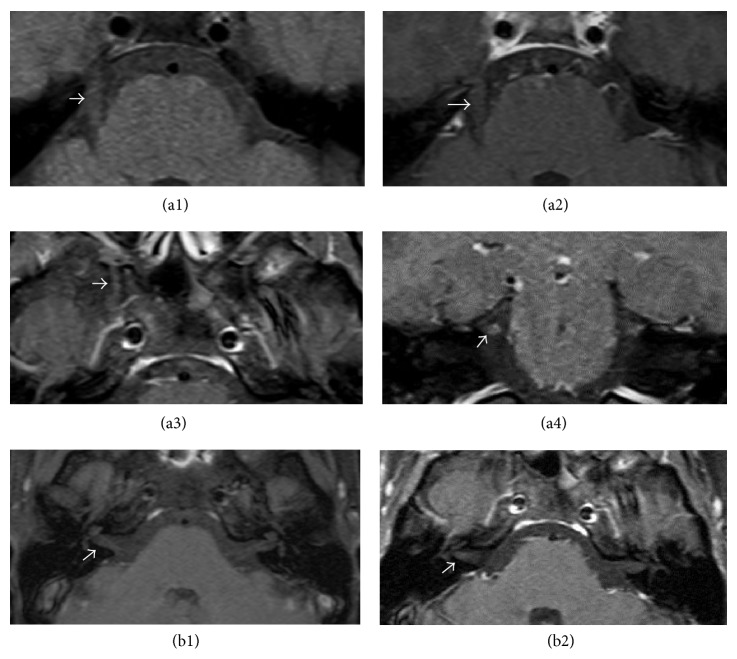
Axial MRI sections of the brainstem at the pontine level demonstrated fusiform enlargement of the fifth cranial nerve at the cisterna level on the T1-weighted images (a1). Postcontrast, fat-suppressed T1 images showed slight enhancement that is disclosed on cisterna level of the fifth cranial nerve (a2) and enlargement of the maxillary division of the fifth cranial nerve with slight enhancement while it passed foramen rotundum (a3). Also coronal MRI section at the level of the fifth cranial nerve showed slight enhancement (a4). Axial MRI sections showed brain stem at the level of facial and vestibulocochlear nerves in precontrast image (b1) and slight enhancement in postcontrast study (b2).
